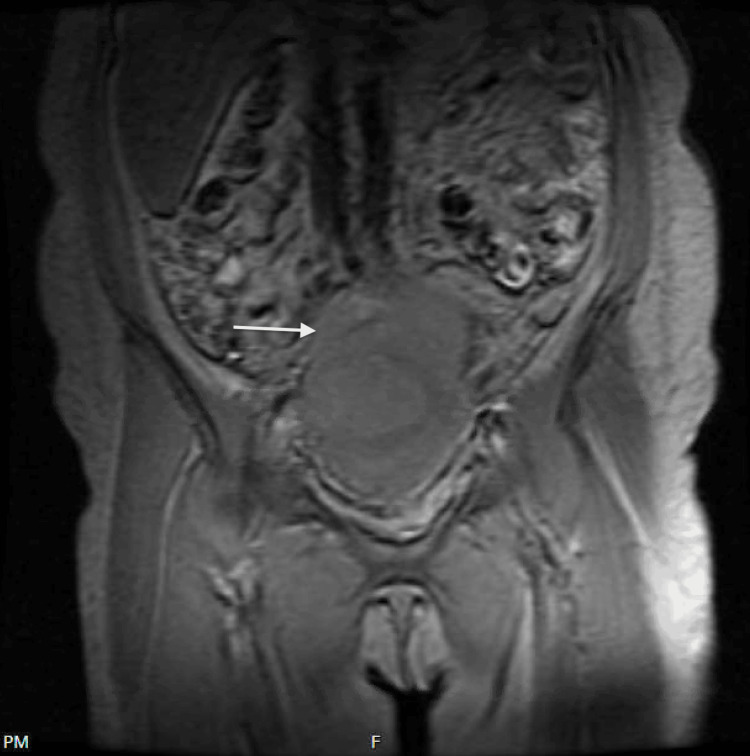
MRI of the pelvis showing a large fibroid uterus. The white arrow shows a mass in the uterus concerning for leiomyosarcoma or leiomyoma. MRI, magnetic resonance imaging
